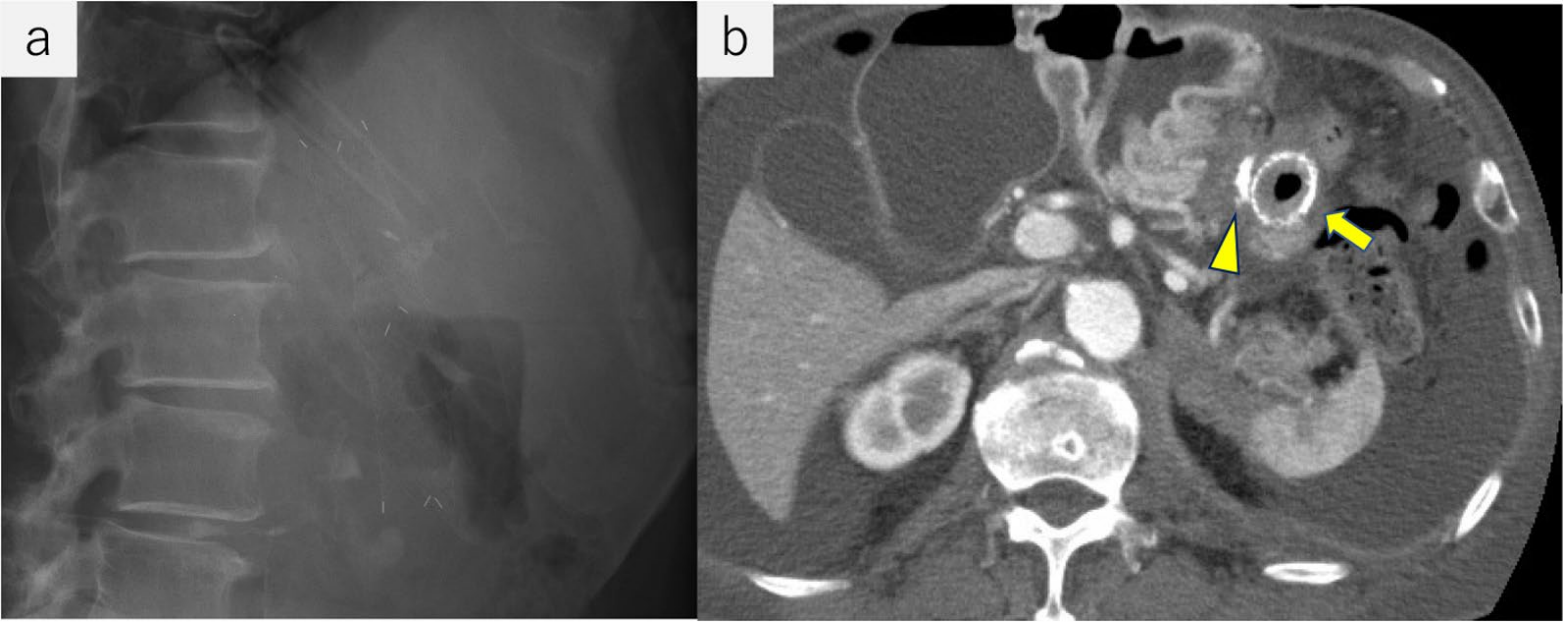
a Plain radiography 47 days after surgery confirmed the stent was open and had not migrated. b Computed tomography 88 days after surgery showed the stent was open and located at the gastrojejunostomy anastomosis (arrow). A staple at the site is visible (arrowhead). However, ascites had increased and the cancer had progressed
